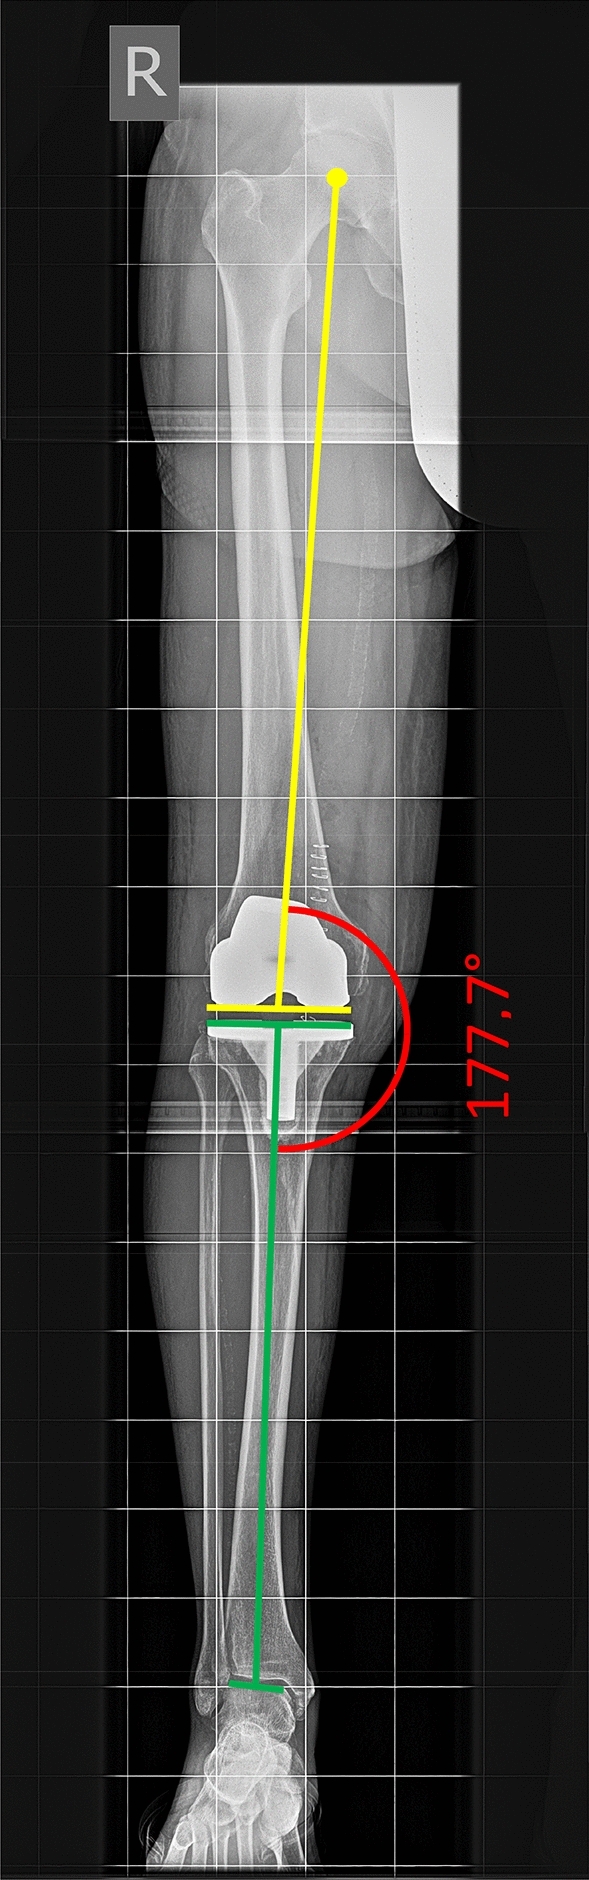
Postoperative measurement of mechanical axis on a long-leg weight-bearing radiograph
